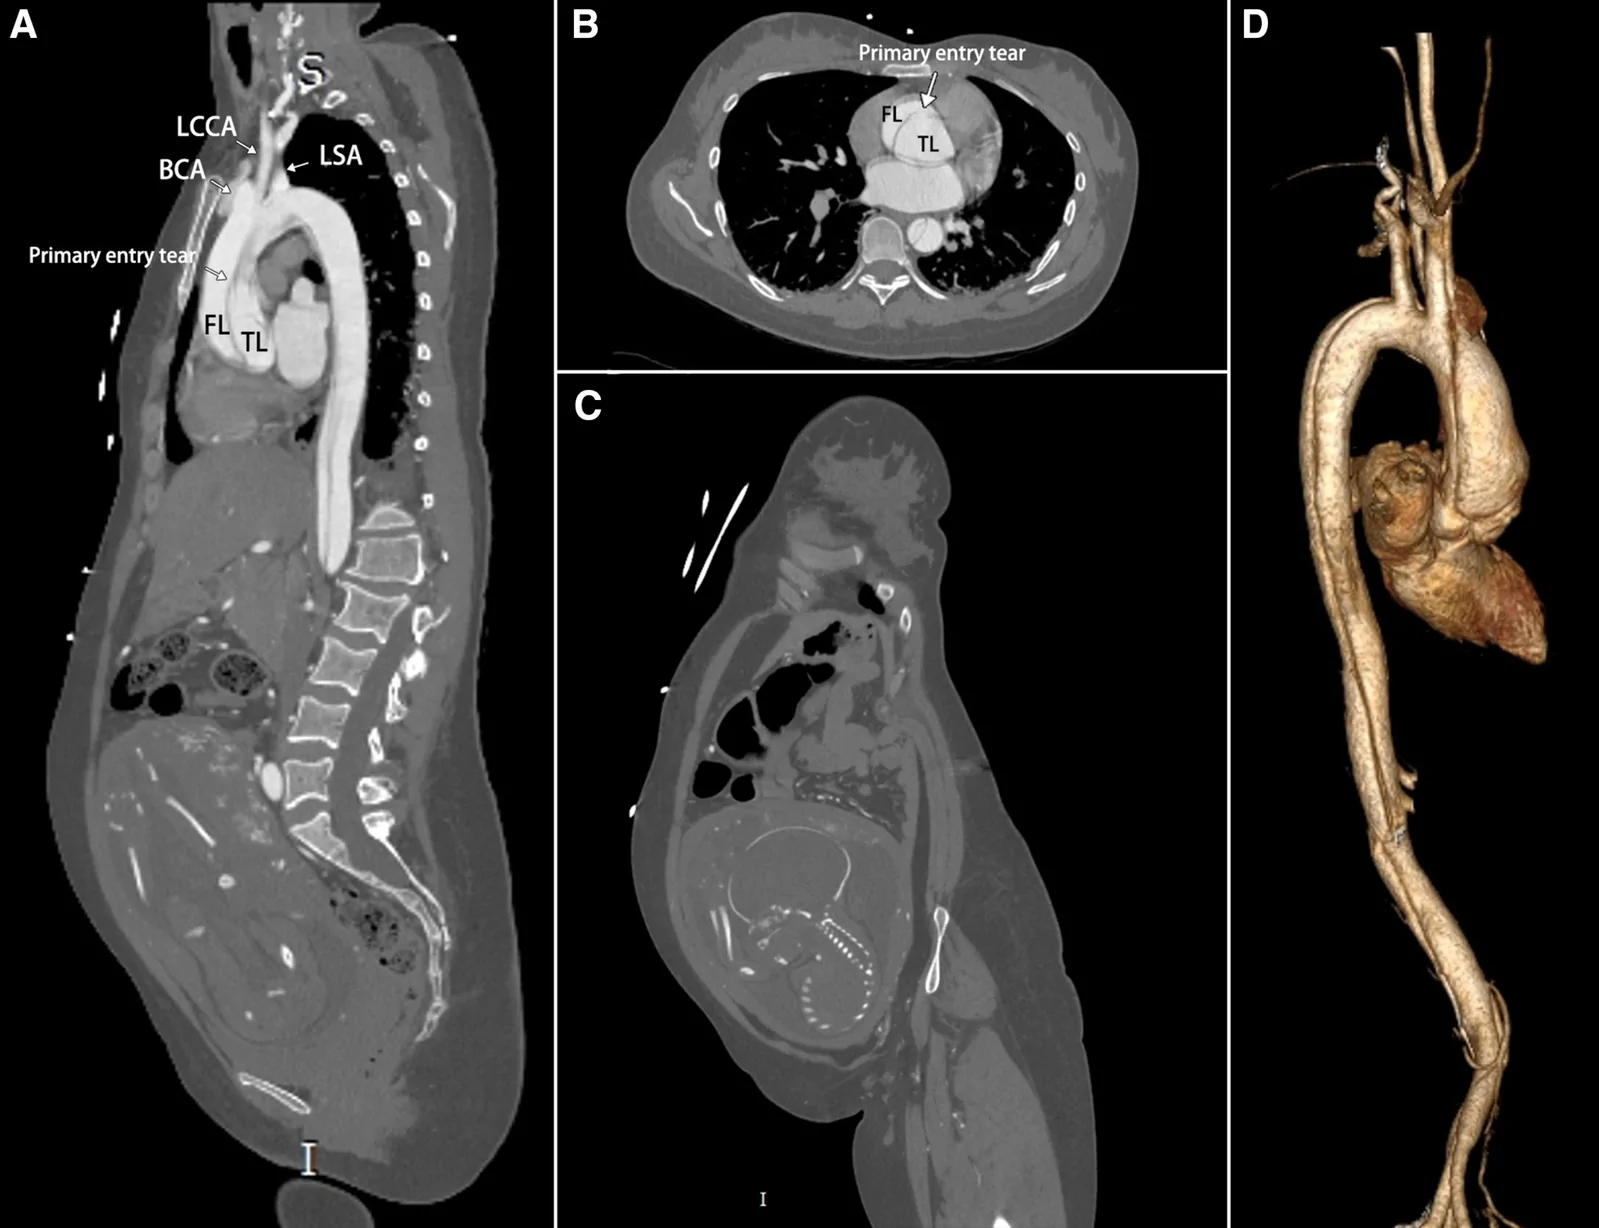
Preoperative computed tomography angiography (CTA) findings in the pregnant patient. (A) Sagittal reformatted CTA image demonstrating acute Stanford type A aortic dissection involving the ascending aorta and aortic arch. The true lumen (TL), false lumen (FL), brachiocephalic artery (BCA), left common carotid artery (LCCA), and left subclavian artery (LSA) are indicated. (B) Axial CTA image showing the TL and FL in the ascending aorta. The arrows in panels (A and B) indicate the site corresponding to the primary entry tear at the aortic sinus, as confirmed intraoperatively. (C) Sagittal CTA image showing the viable fetus in utero at 29 weeks and 3 days of gestation. (D) Three-dimensional volume-rendered CTA reconstruction demonstrating the extent of the aortic dissection. BCA = brachiocephalic artery, FL = false lumen, LCCA = left common carotid artery, LSA = left subclavian artery, TL = true lumen.
